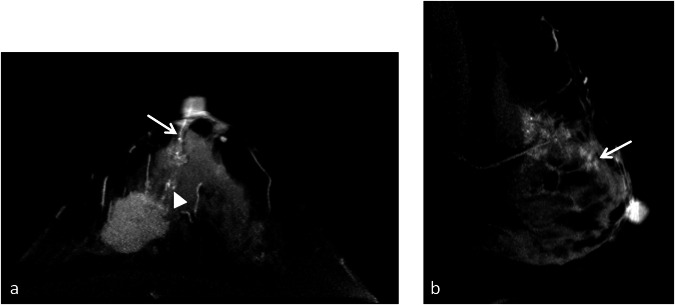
Examples of TNE within 2 cm of the NAC. a A 40-year-old patient with invasive ductal carcinoma in the left breast. An axial CE-CBBCT image showed examples of TNE extending to the NAC (arrow) and suspicious calcification within 2 cm (arrowhead). A mastectomy was performed, and NAC involvement was detected in pathology. b A 50-year-old patient with invasive ductal carcinoma in the left breast. A sagittal CE-CBBCT image showed an example of TNE non-extending to the NAC but within 2 cm (arrow). A mastectomy was performed, and NAC involvement was not detected. NAC, nipple–areolar complex; CE-CBBCT, contrast-enhanced cone-beam breast CT; TNE, tumor–nipple enhancement.
